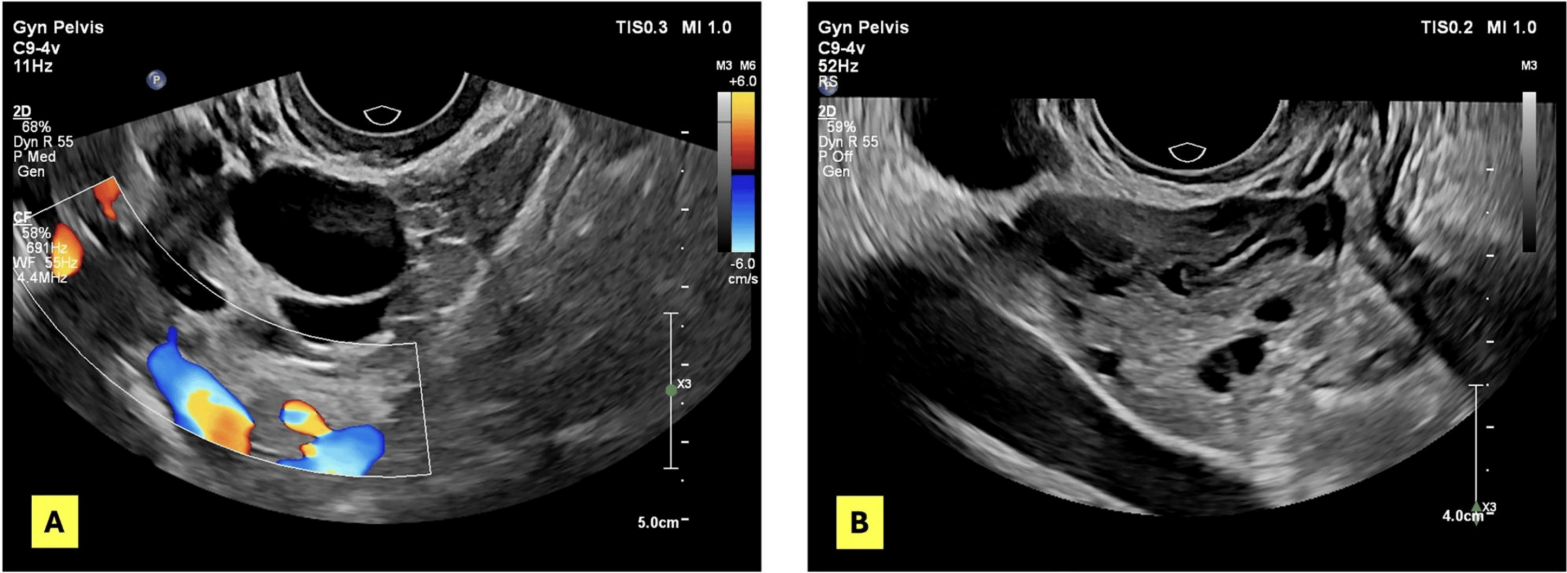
Representative transvaginal ultrasonographic ovarian morphology. Representative transvaginal ultrasonographic images showing a normal ovary in a control subject (A) and polycystic ovarian morphology in a patient with PCOS (B). PCOS: polycystic ovary syndrome
